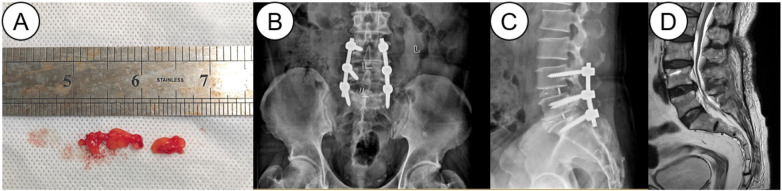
(A) Fat tissue obtained intraoperatively from the spinal canal; (B) and (C) display postoperative lateral and anteroposterior x-rays of the lumbar spine fixation; and (D) depicts a postoperative lumbar spine MRI T2 sequence.
